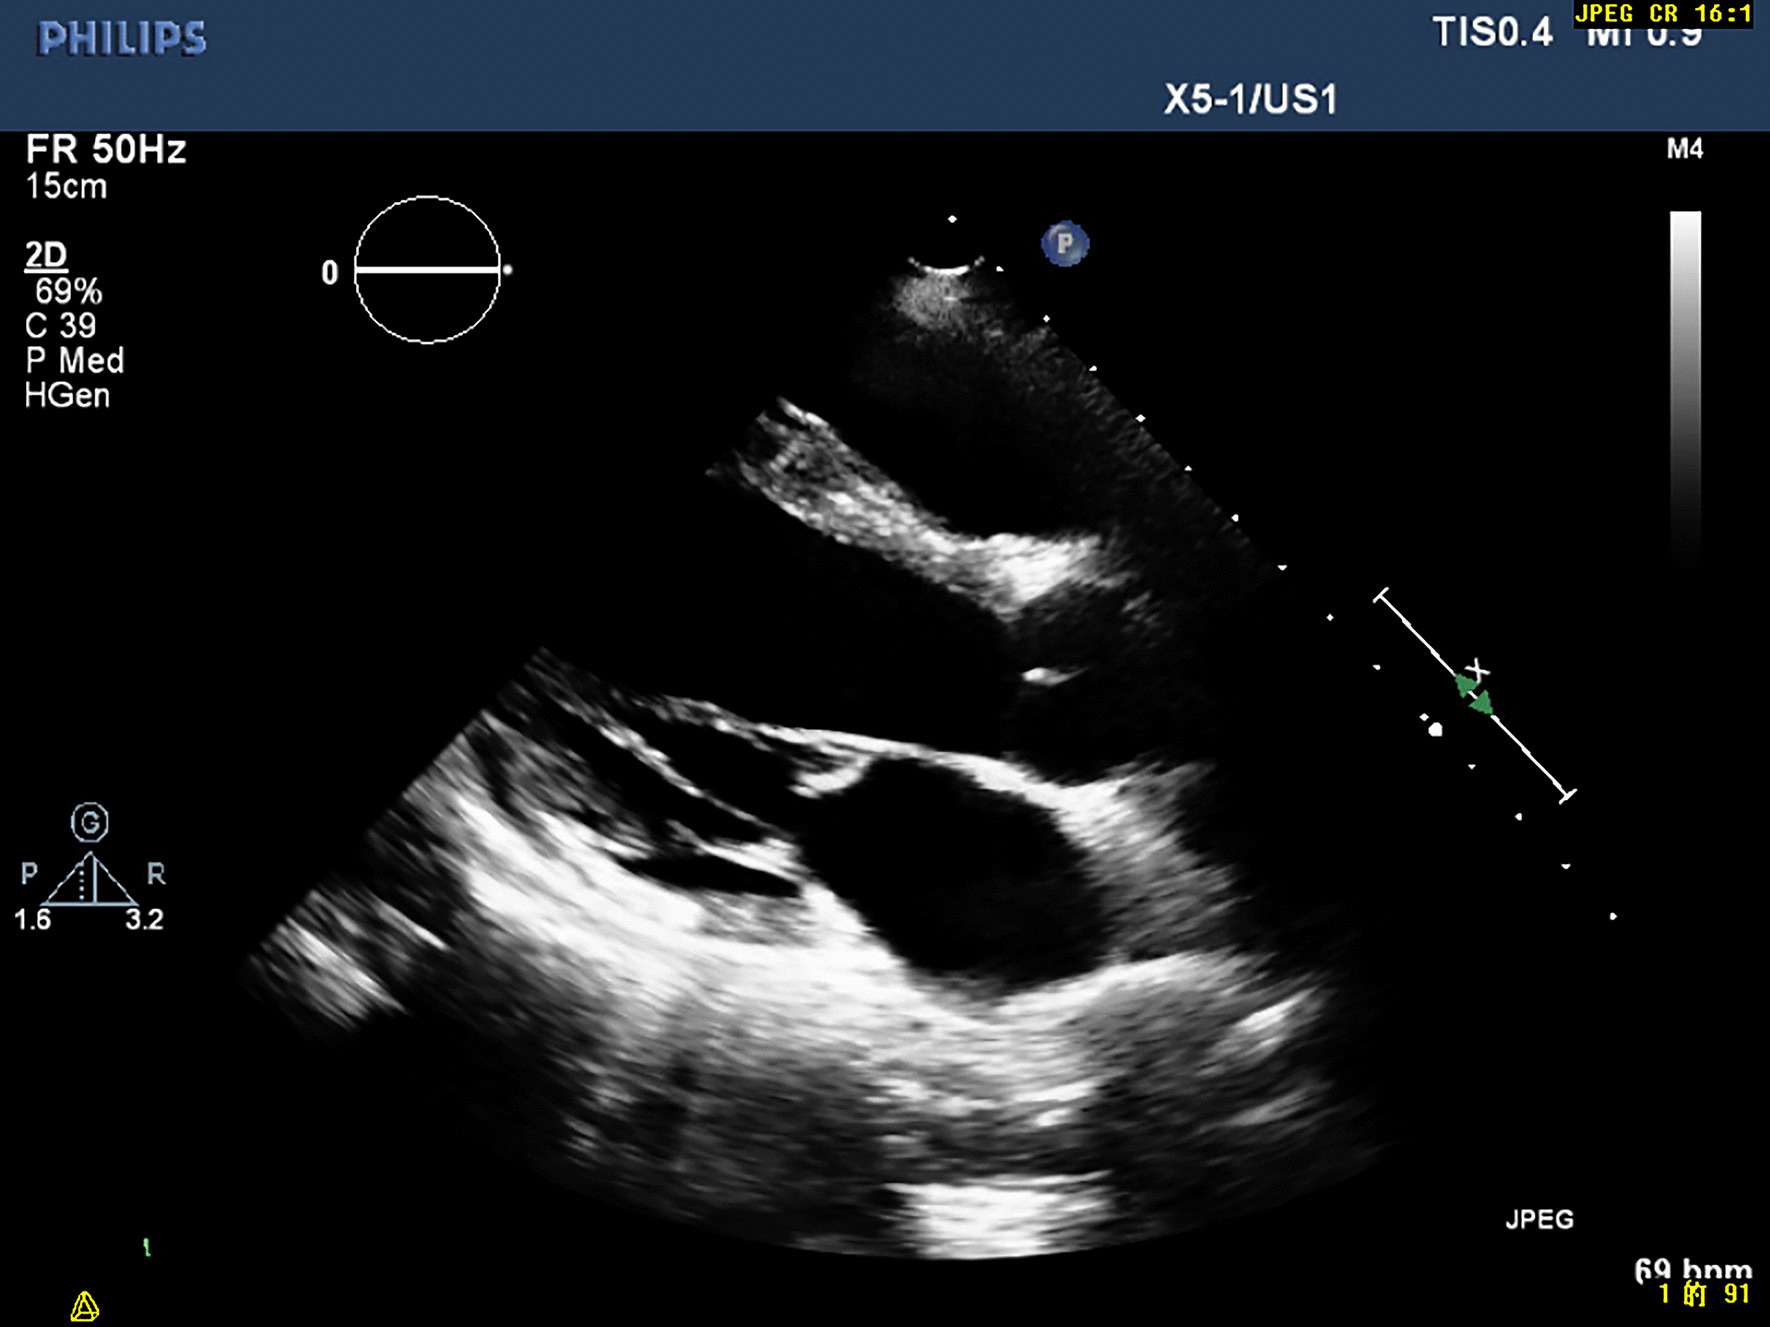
The parasternal long axis views of the left ventricle before coronary angiography
